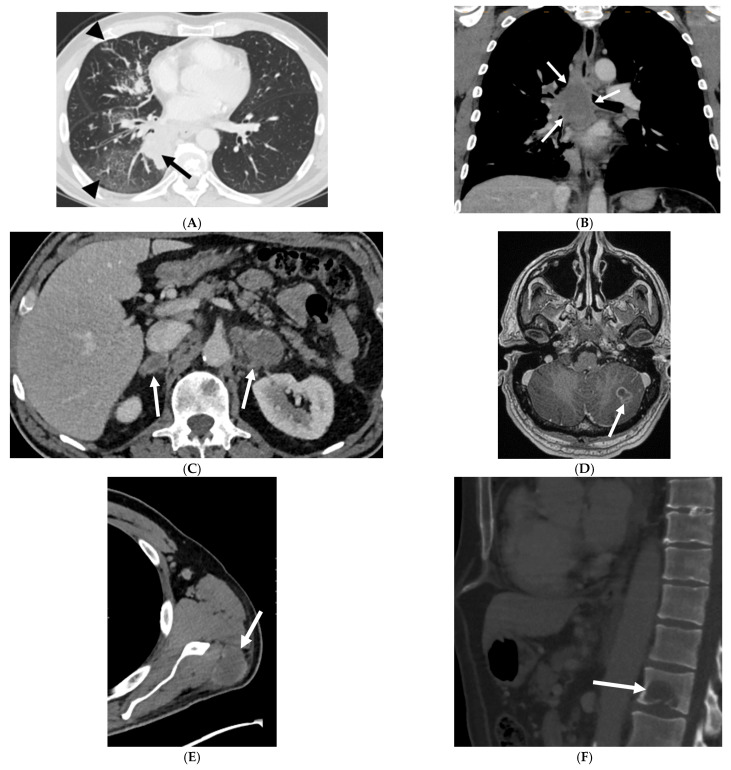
Representative imaging features in a 64-year-old male prior smoker with G12C KRAS NSCLC. Pretreatment CT images show a solid mass in the right lower lobe ((A), black arrow) and associated septal and peribronchial thickening consistent with lymphangitic carcinomatosis ((A), black arrowheads). There was extensive mediastinal and hilar lymphadenopathy ((B), white arrows), bilateral adrenal metastases ((C), white arrows), brain metastasis ((D), white arrow), soft tissue metastasis ((E), white arrow), and a lytic osseous metastasis of the first lumbar vertebral body ((F), white arrow).
